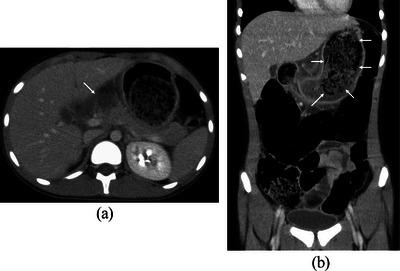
Computed tomography findings. (a) Axial computed tomography showed a non‐enhancing lesion (arrow) in the pancreatic head. (b) Coronal computed tomography showed a large intra‐gastric mass (arrows) with numerous small air bubbles.
